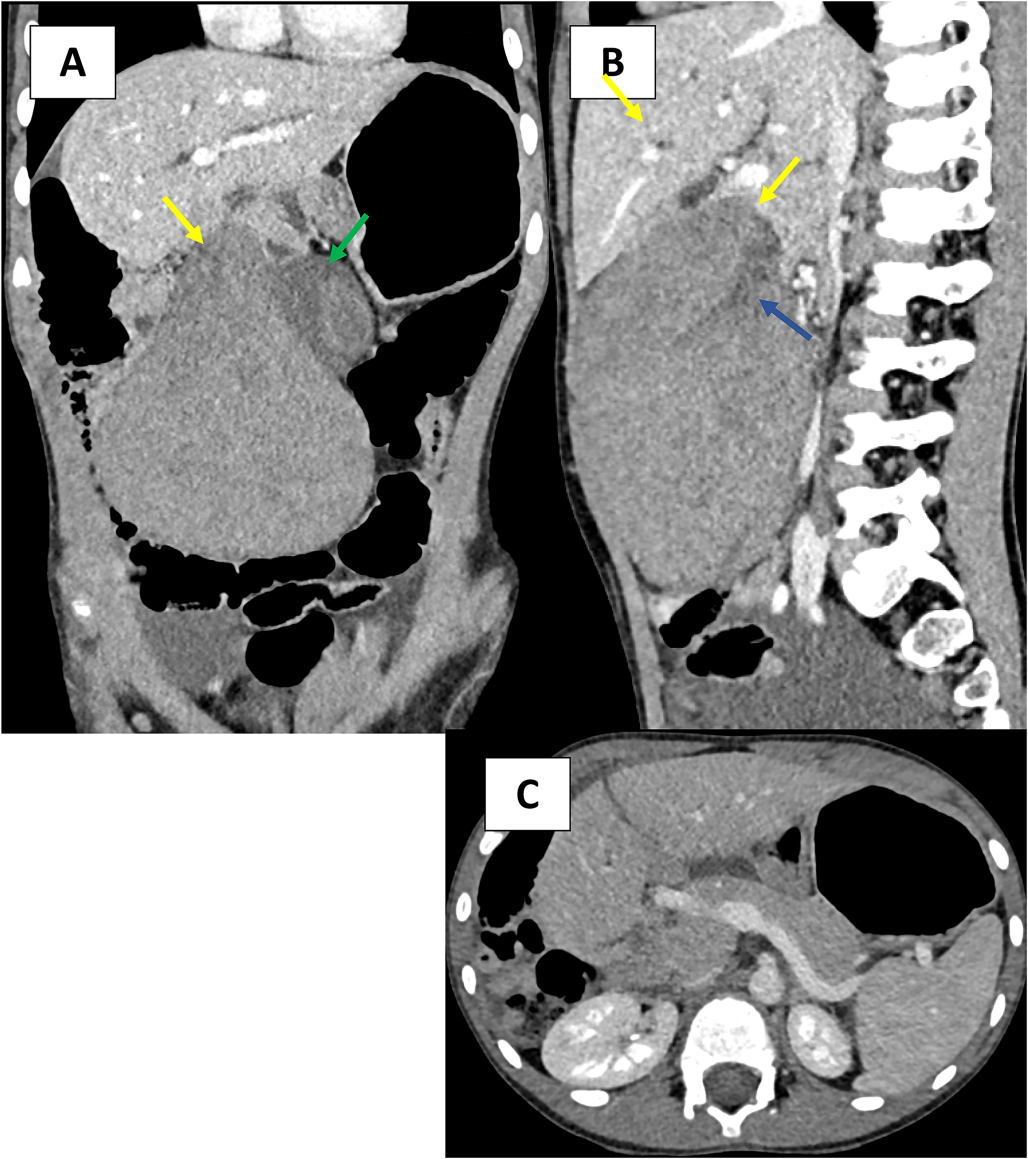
(A–C) Contrast-enhanced, axial, coronal, and sagittal CT images in the venous phase demonstrate a large, heterogenous low-attenuation mass in the right abdomen. (A–C) The mass shows tubular structures, likely a thrombosed portal vein (blue arrow) extending into the thin pedicle of the torsed ALL (yellow arrow). The gallbladder is displaced to the left and with a thick wall (green arrow).
